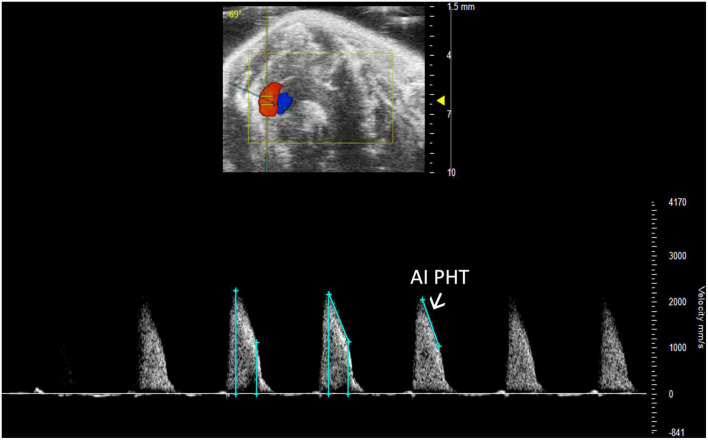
Aortic insufficiency pressure half time (AI PHT) represents the degree of regurgitation and reflects the left ventricular end diastolic pressure and is measured in pulsed wave (PW) Doppler mode of the aortic valve.
